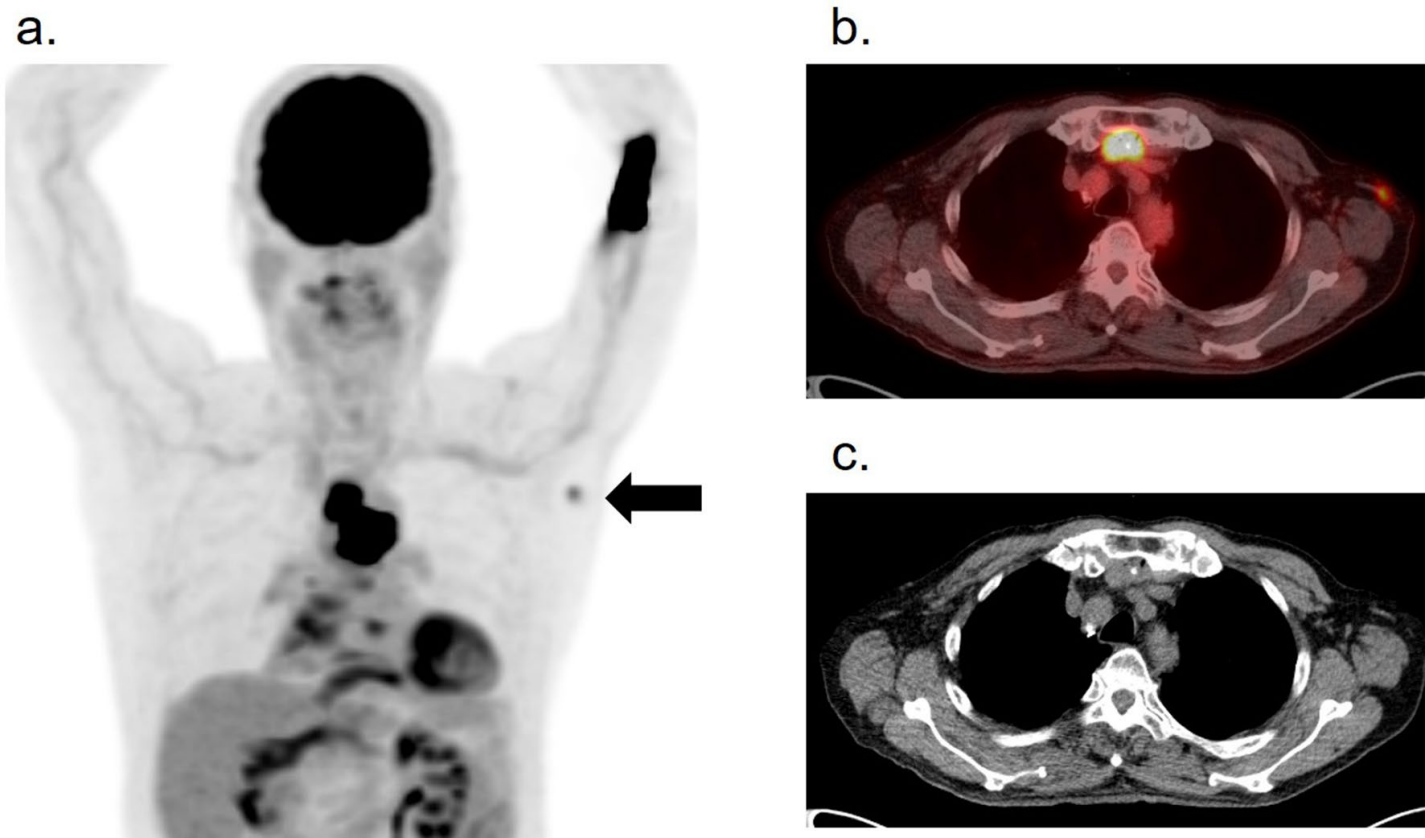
Patient with retrosternal-reconstructed esophageal cancer. A hot spot at the left axillary lymph node as a result of tracer extravasation in the ipsilateral arm is visualized. a MIP image, b axial section of the fusion image. The CT shows a normal lymph node configuration (c)
